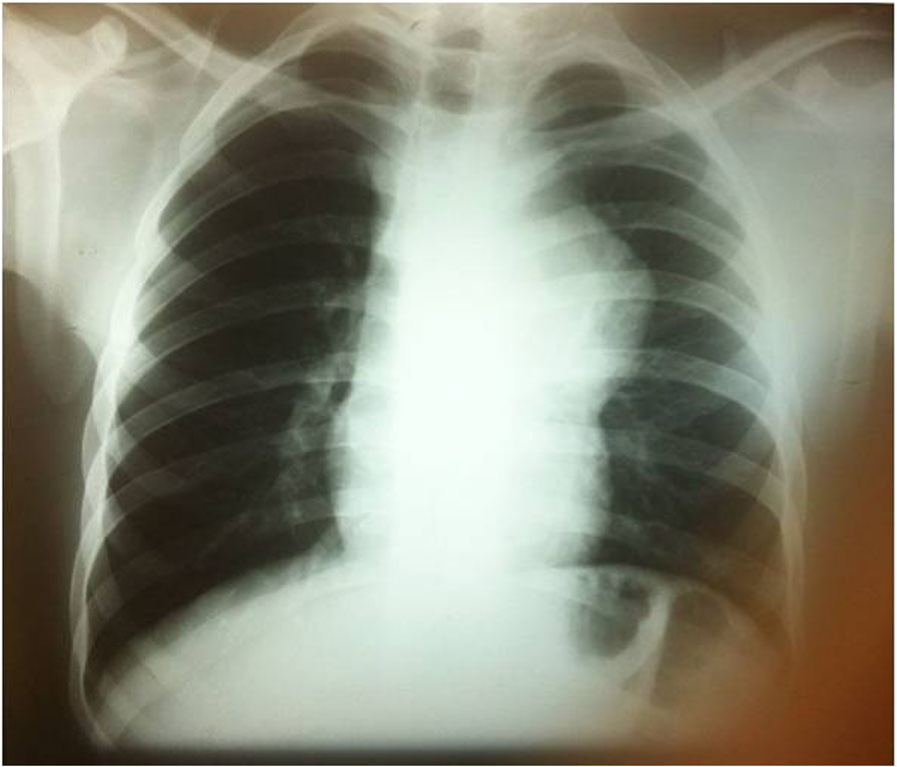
Posterior-anterior chest X-ray demonstrating mediastinal widening
